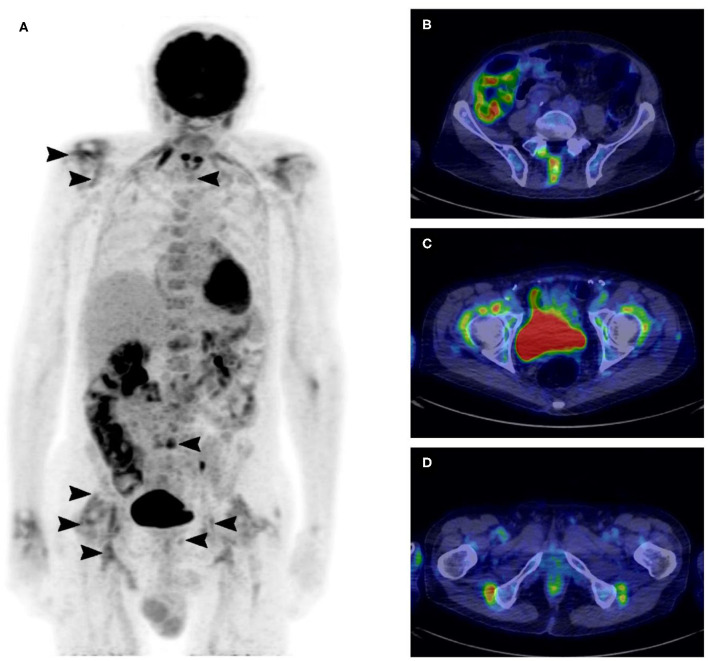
^18^F-FDG PET/CT maximum-intensity projection showing uptake at the 17 sites of the skeleton in a patient suffering from polymyalgia rheumatica (A), axial fused ^18^F-FDG PET/CT with typical uptake of polymyalgia rheumatica at the lumbar interspinous bursa (B), at the iliopectineal bursa (C), and at the ischial bursa (D).
